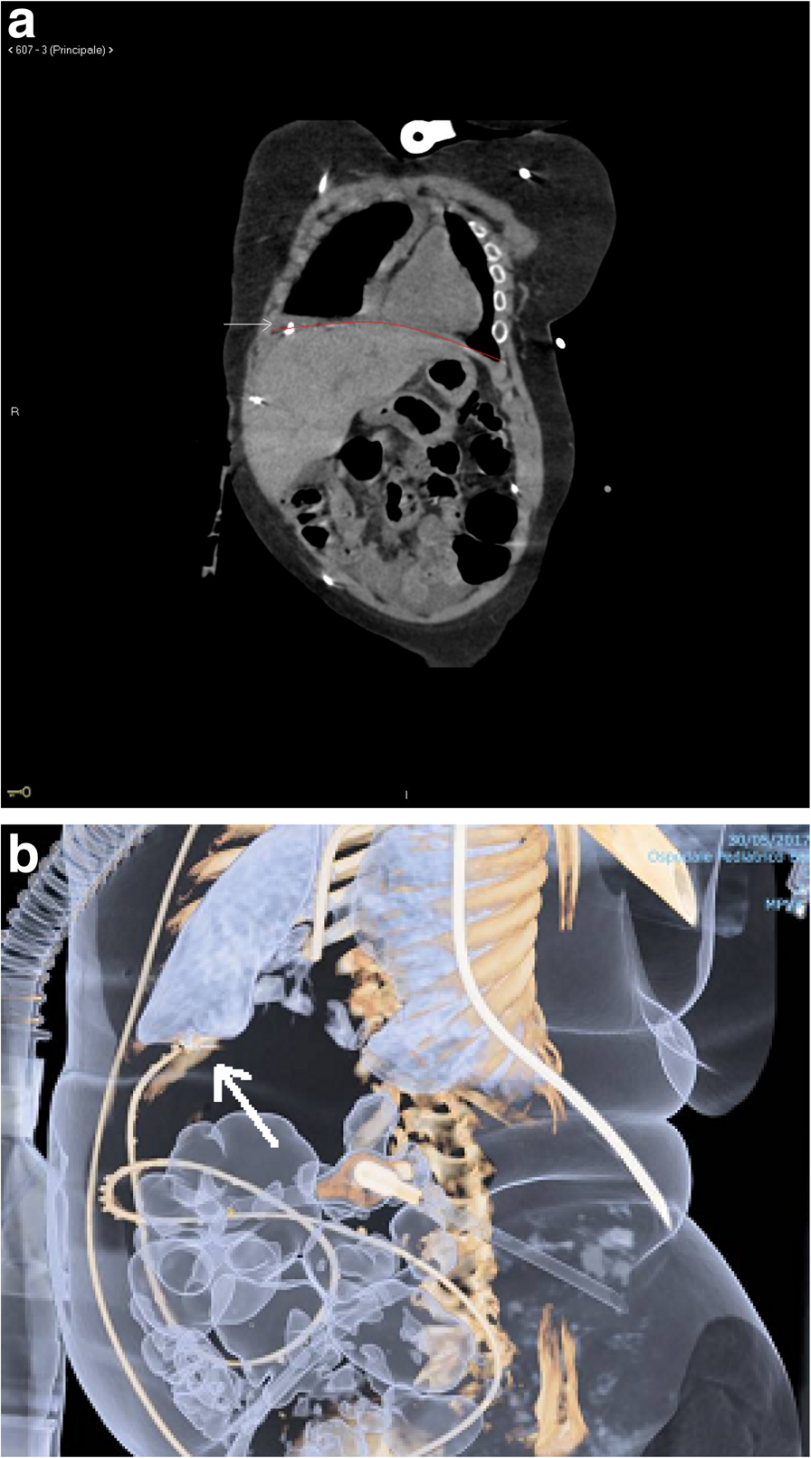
a Coronal plane of chest and abdomen CT scan demonstrating the dislocation of the distal end of VP shunt situated over the diaphragmatic cupola and within the pleural cavity. b Abdomen CT scan 3D reconstructions
